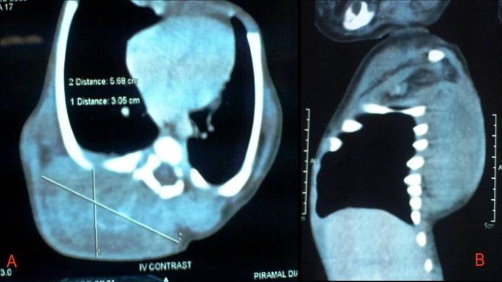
(A) & (B) (Transverse and Sagital views): CT-scan chest and upper abdomen of a newborn who presented with a hard mass on his back of chest, showing a minimally enhancing low-attenuating soft tissue mass, localized in posterior chest wall in right para-vertibral and right intra-scapular region.
